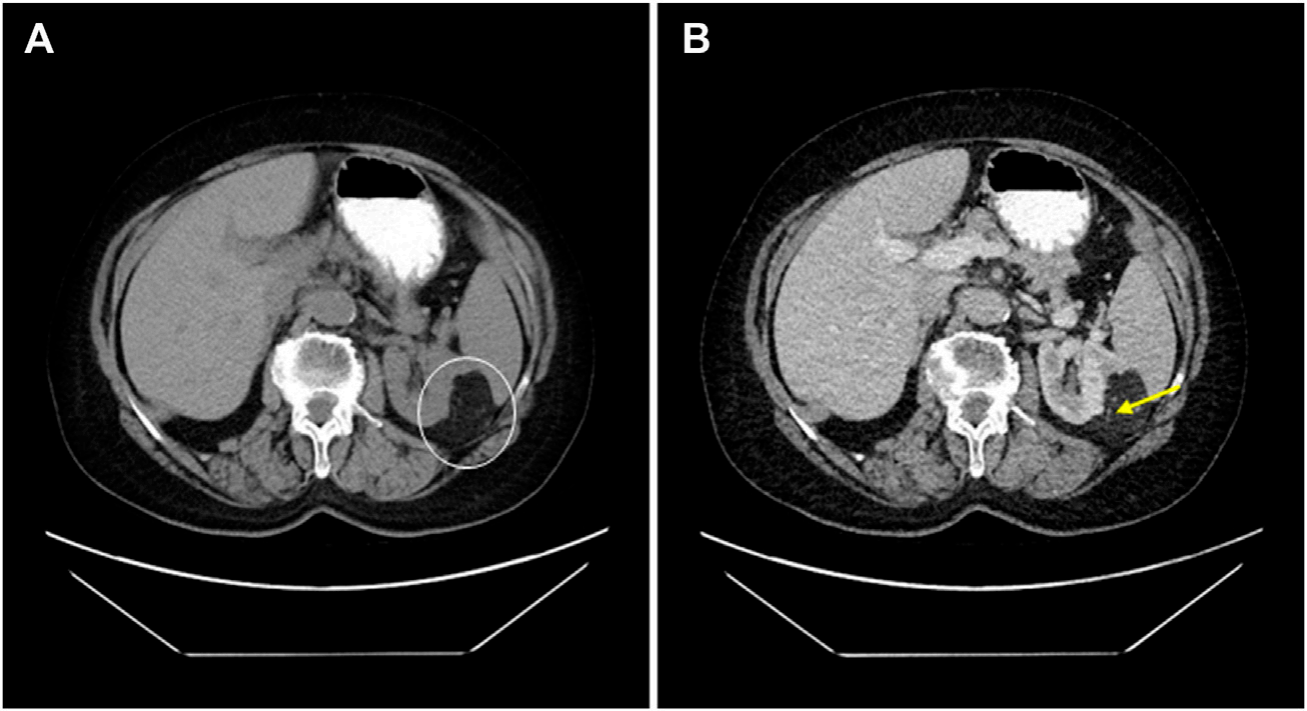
68-year-old female patient’s CT scan accidentally reveals an angiomyolipoma. (A) A native CT scan shows a lobulated 54 mm maximal axial diametric mass with a mean density of -79 HU (white circle). (B) In the same plane, the venous phasic cross-section shows that the difference does not change substantially; moreover, the thick-wall blood vessels become visible (yellow arrow).
